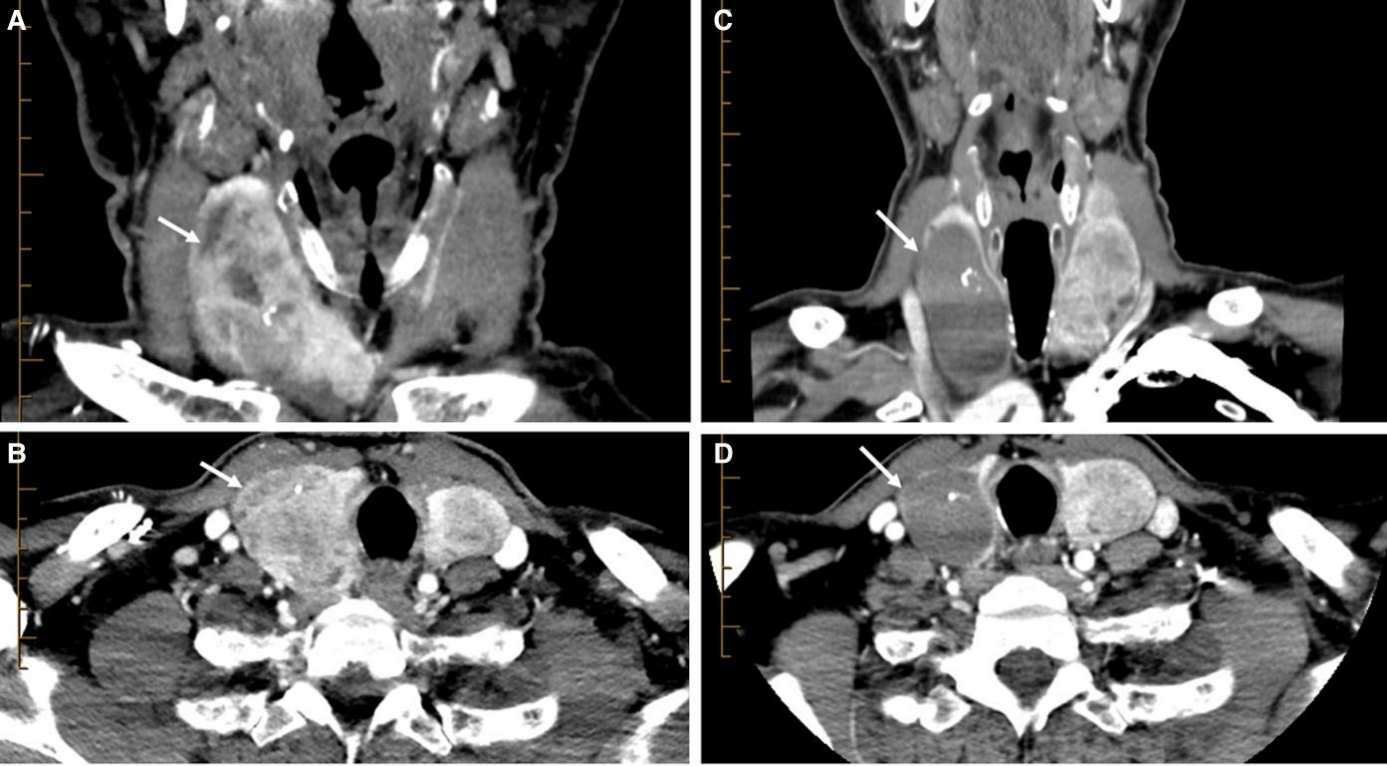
Toxic nodule case. A 68-year-old man presenting with tachycardia and anxiety secondary to hyperthyroidism. Thyroid uptake scan confirmed increased uptake in the right lobe of the thyroid. A, Coronal and B, axial computed tomography (CT) of the neck with contrast pre procedure demonstrates a significantly enlarged right thyroid lobe. Following embolization, C, coronal and D, axial CT of the neck with contrast post procedure demonstrates complete absence of enhancement of the right thyroid lobe, corresponding with glandular necrosis. This patient is currently euthyroid.
